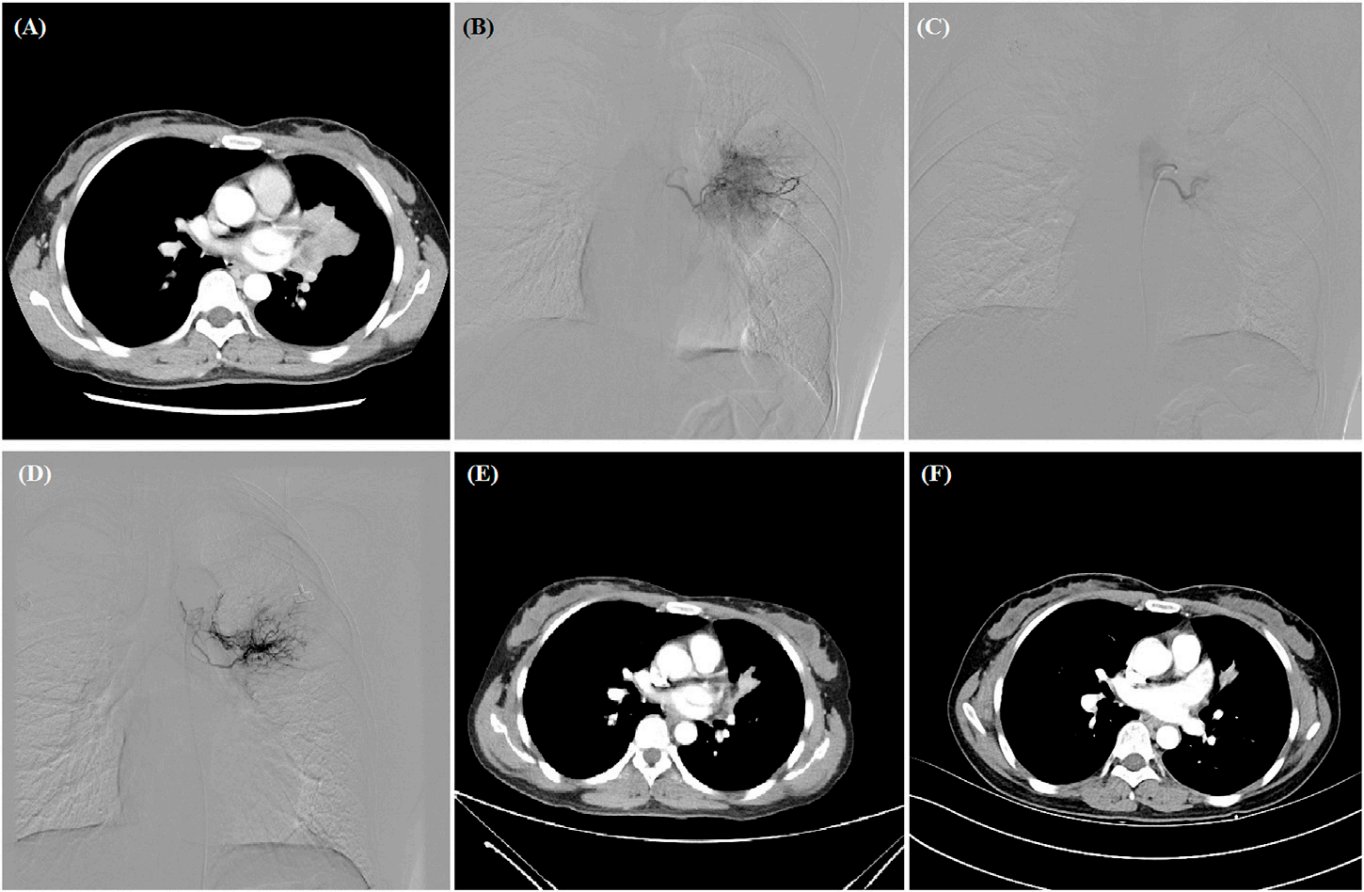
A 38-year woman with advanced adenocarcinoma treated by DEB-TACE. (A) CT examination revealed central malignant tumor of left lung. (B,C) The left bronchial artery catheterized and the blood supply artery was embolized by oxaliplatin-loaded CB. (D) The second DEB-TACE was performed after 2 months. (E,F) The left lung tumor was found to shrink at 1- and 3-month follow-up, and this woman is still alive after 36.3 months.
